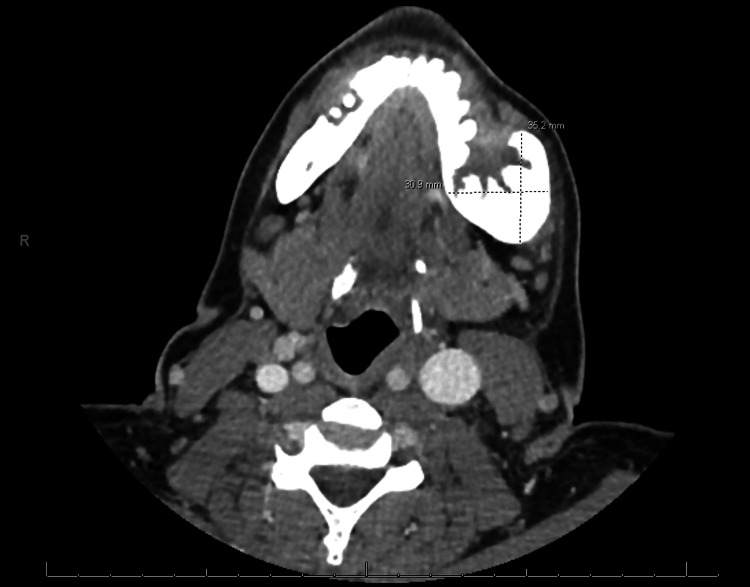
Axial CT with contrast showing an solid expansile mass centered in the left mandibular body now measuring approximately 3.5 x 3.1 cm (AP by TV) with similar local mandibular destruction and heavily calcified components primarily within the posterior and inferior aspect of the lesion AP: anteroposterior; TV: transverse
